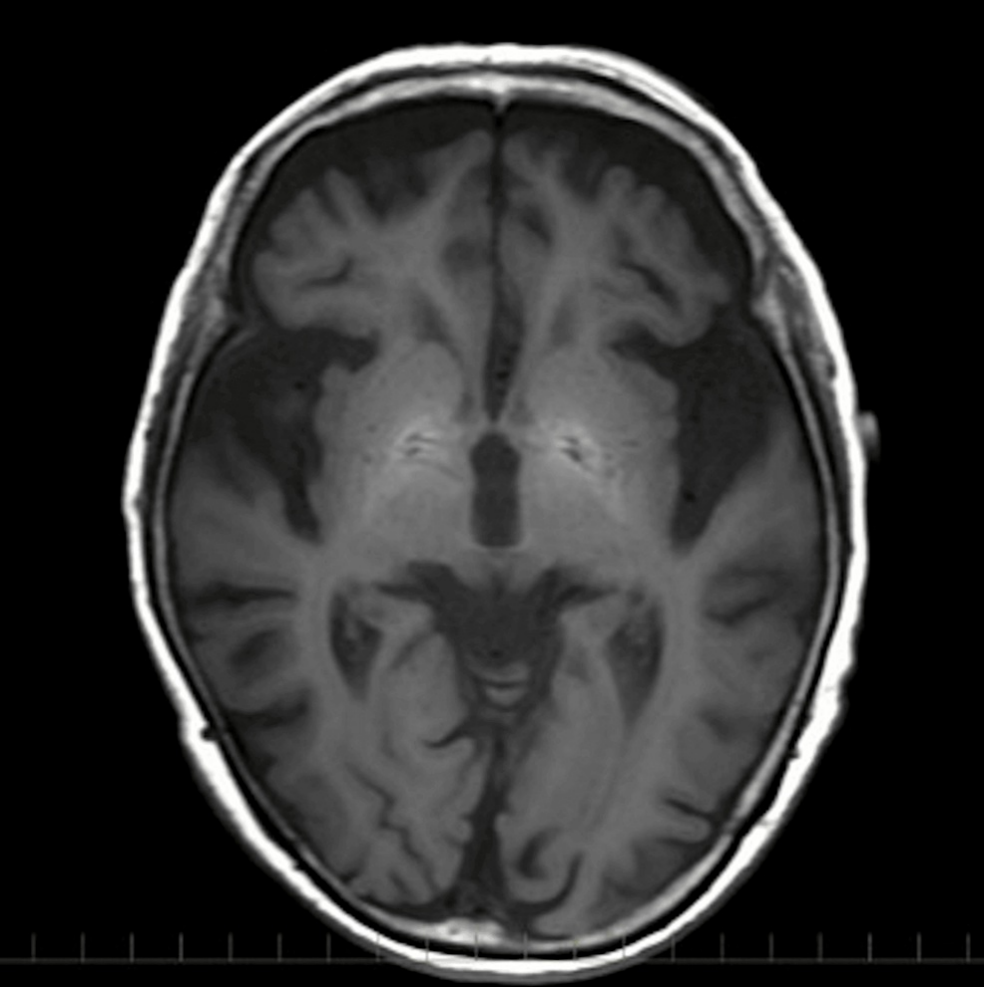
Axial T1-weighted MRI Axial T1-weighted MRI showed a markedly high-intensity area in the globus pallidum.
